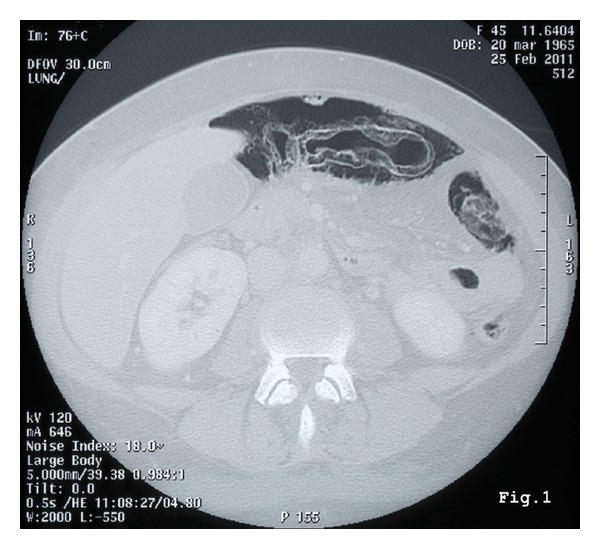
CT of the abdomen, signs of pneumoperitoneum, and pneumatosis.
